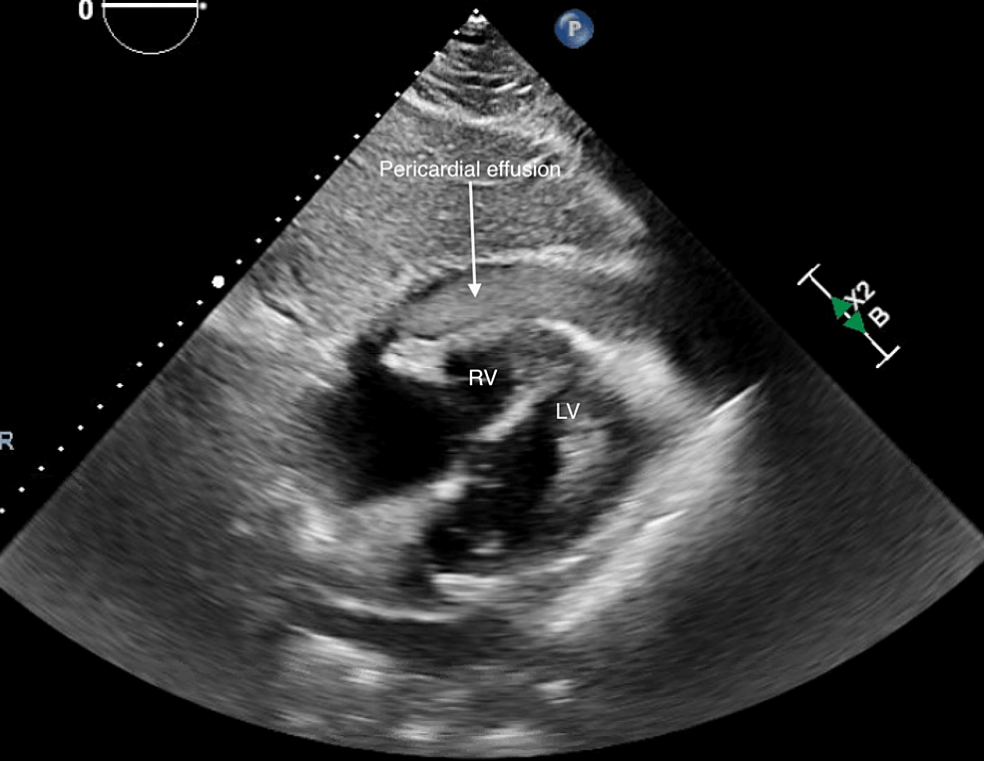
Transthoracic apical echocardiogram demonstrating a hyperdense pericardial effusion, as indicated by the arrow in the figure. LV: left ventricle, RV: right ventricle.
